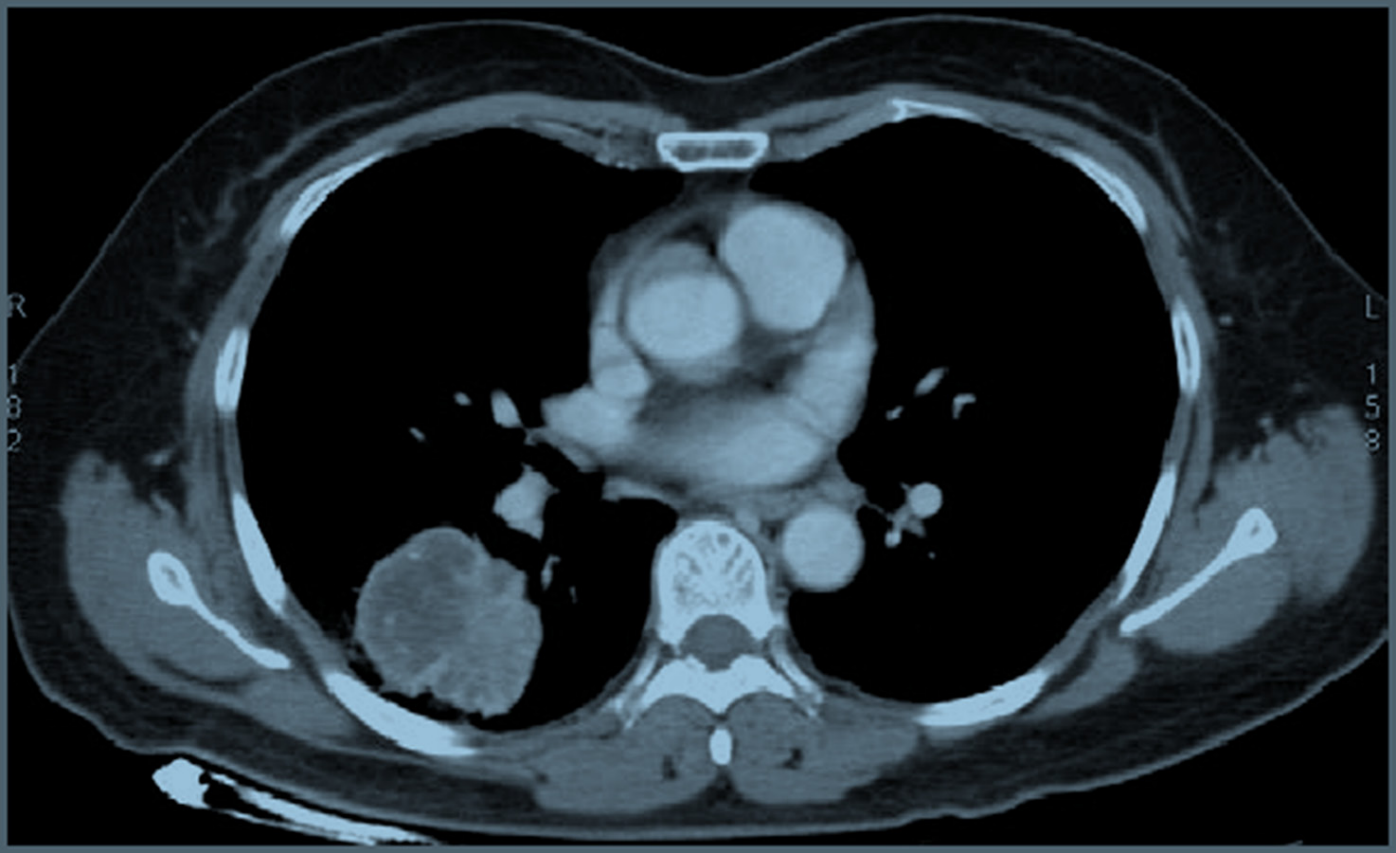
CT scan showing the mass in the right lower lung
